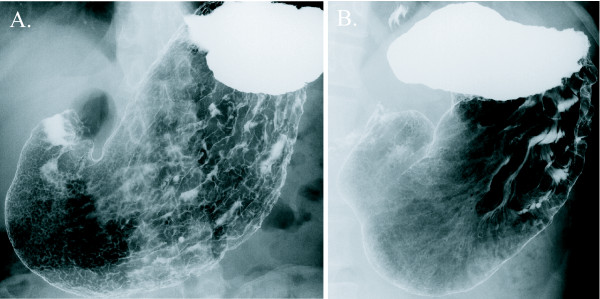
The typical two types of chronic gastritis observed in the double-contrast barium X-ray images. (A) Atrophic gastritis with enlarged areae gastricae. (B) Hypertrophic gastritis with thickened folds on the greater curvature.
